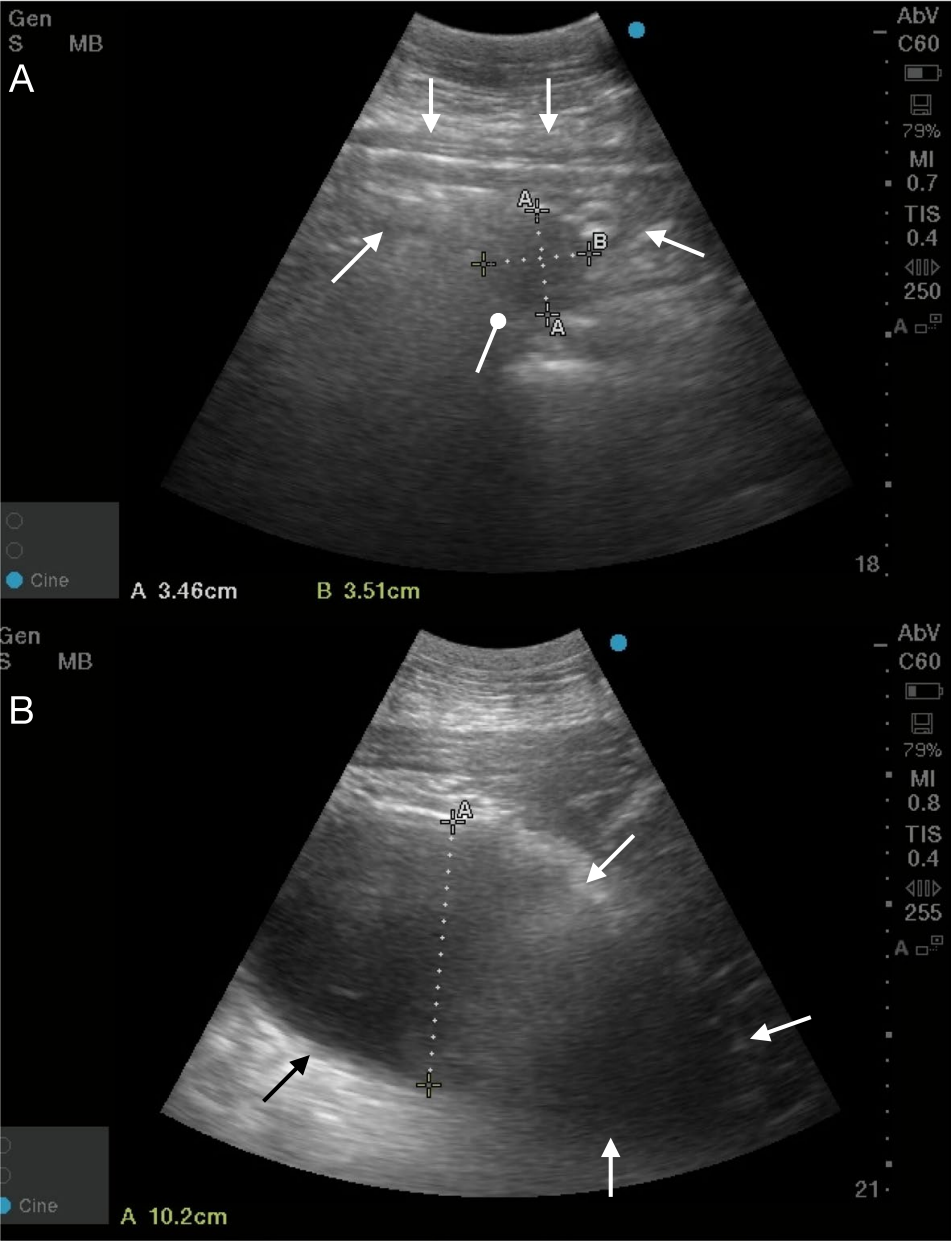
Ultrasound images during false pregnancy (FP) in a walrus. A Corpora luteal (round headed line) of false pregnancy shown within dotted crossed lines. The border of the ovary is highlighted by white arrows. B Fluid identified within the uterine horn — dilated to 10.2 cm of a FP walrus. The uterine horn is outlined by 3 white and 1 black arrows
